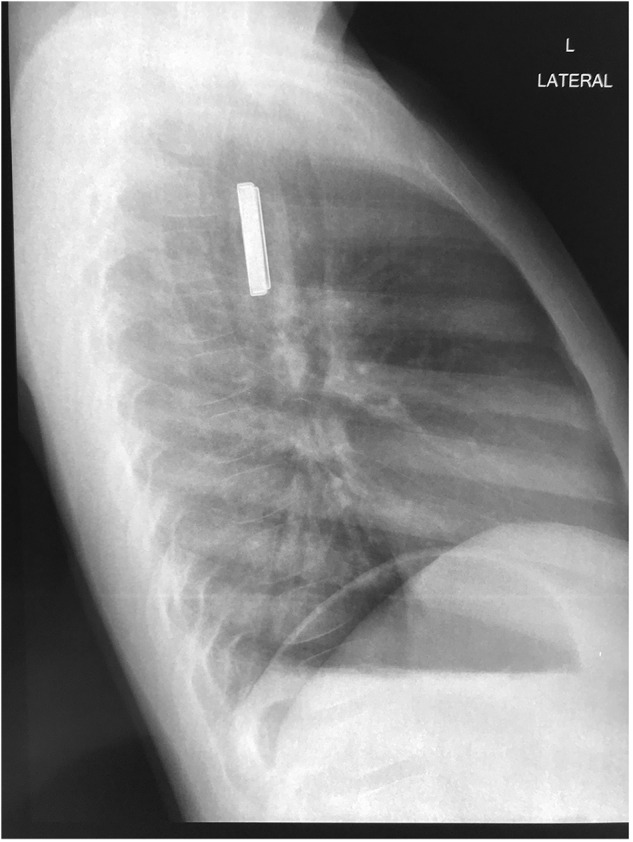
Step-off sign on lateral view chest x-ray.^20^
